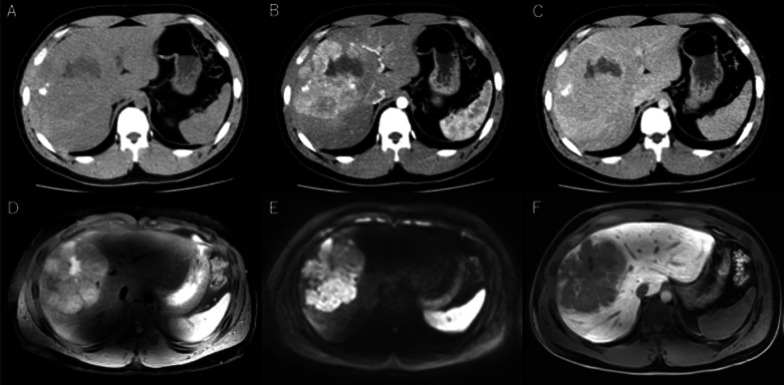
Dynamic computed tomography (CT) and gadoxetic acid-enhanced magnetic resonance imaging. A lobulated mass is visualized located in the anterior sector of the liver. The tumor was seen as a low-attenuating lesion in unenhanced images (A) and as a heterogeneous hyperattenuation in the arterial phase of dynamic CT (B). Hyperattenuation in the arterial phase suggests hypervascularity. The tumor demonstrated washout in the delayed phase of dynamic CT (C). The central scar showed a gradually enhancing pattern with calcification. The scar was seen as a hypointensity on T2WIs (D) and as a marked hyperintensity on DWIs (E). The tumor was visualized as a low signal intensity in the hepatobiliary phase, without gadoxetic acid uptake. Also, the tumor was observed as having a lobulated appearance in the hepatobiliary phase (F)
